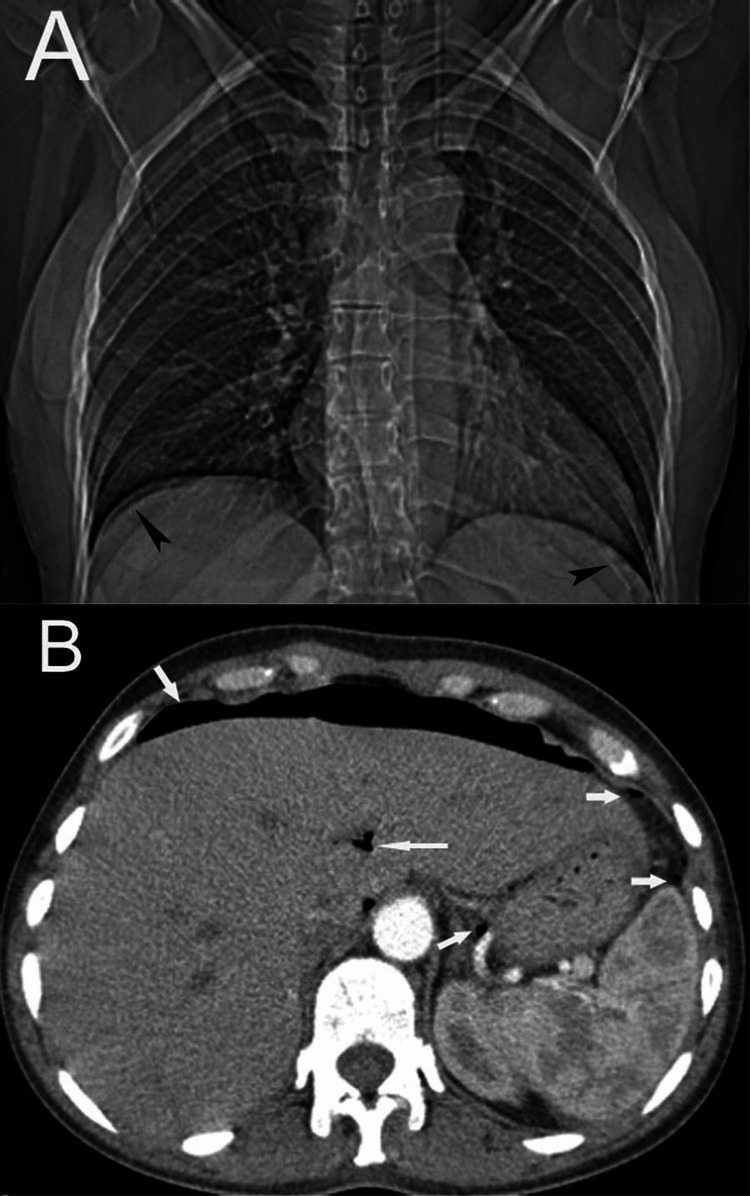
Scans of a 48-year-old female who developed pneumoperitoneum (arrows) during hysterectomy A: The frontal chest radiograph reveals subdiaphragmatic free air (arrowheads); B: An axial CT image of the abdomen displays free peritoneal air (arrows). Free air is also visualized in the biliary tract (long arrow).
